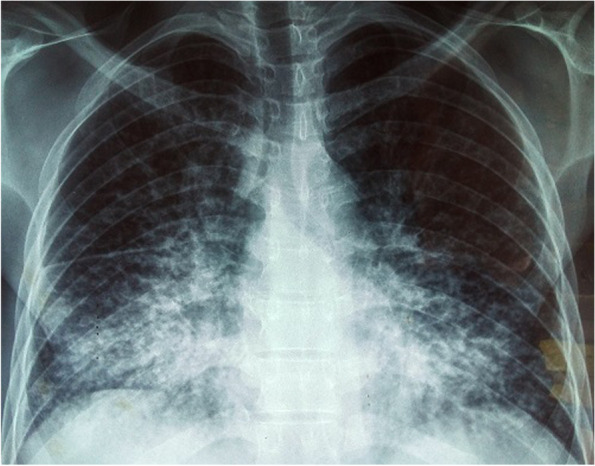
Front view chest X-ray showing reticular-micronodular opacities in the hilar and basal pulmonary regions
